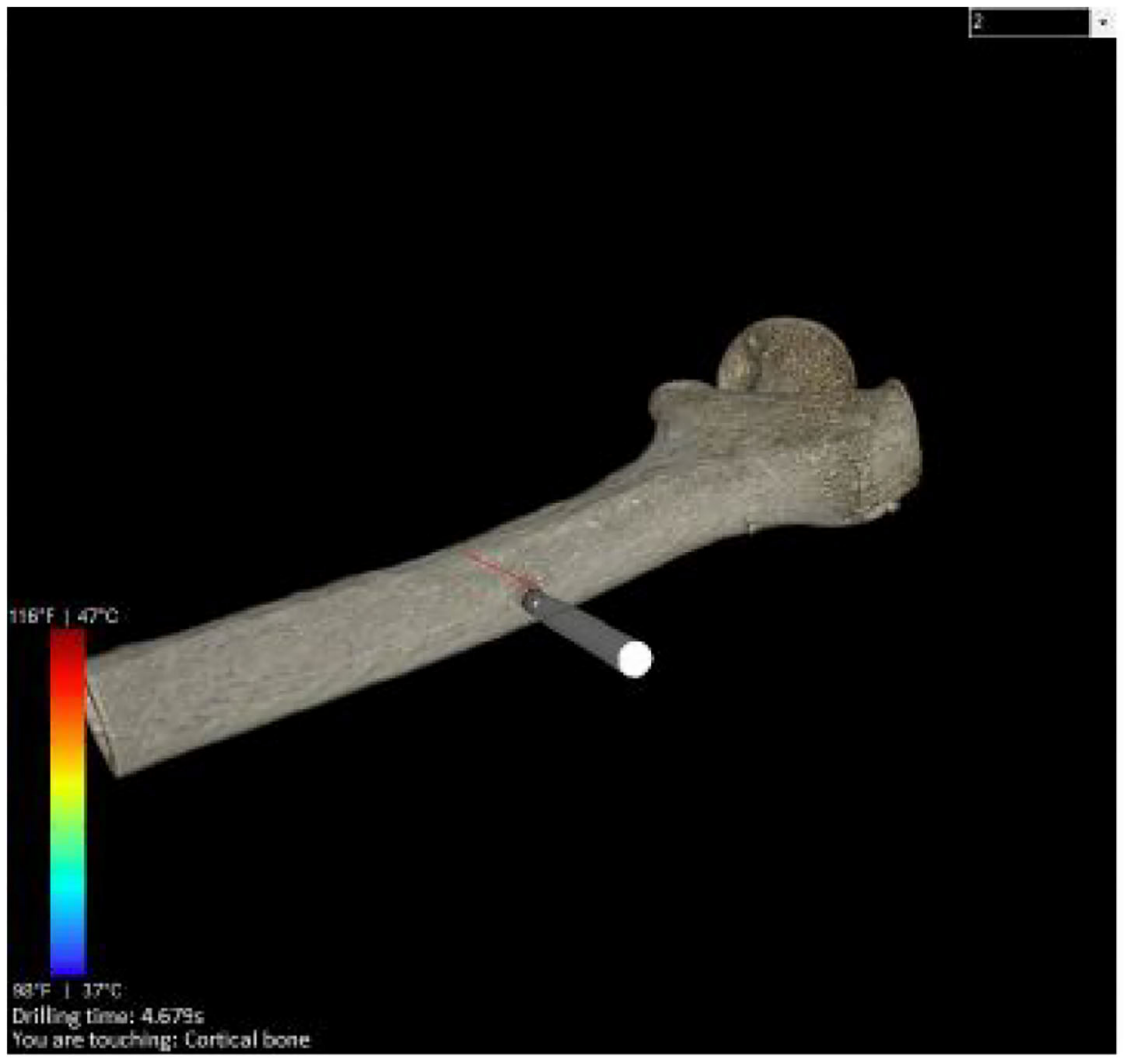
The image shows a rendered bone obtained from the CT data. The user is able to touch or drill the shape, visually by moving the object sticked the bone.
